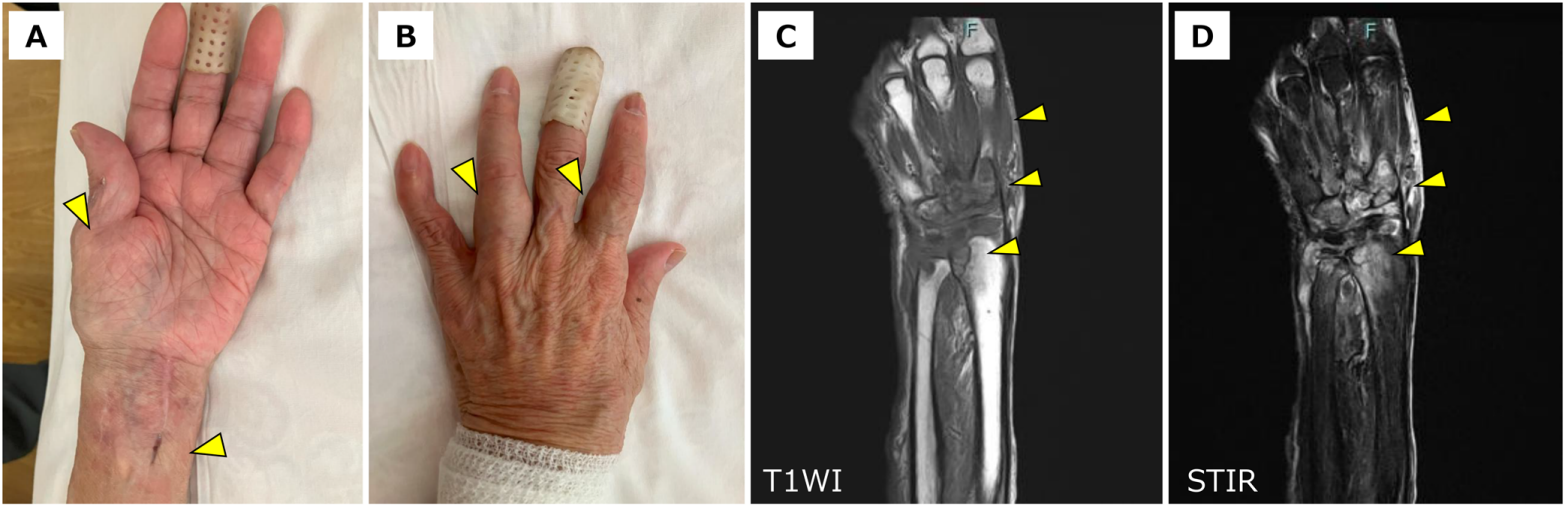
(A, B) Gross appearance of the left forearm demonstrating significant peridigital swelling. (C, D) MRI findings showing medullary osseous edema with concomitant periarticular soft tissue inflammatory changes.
